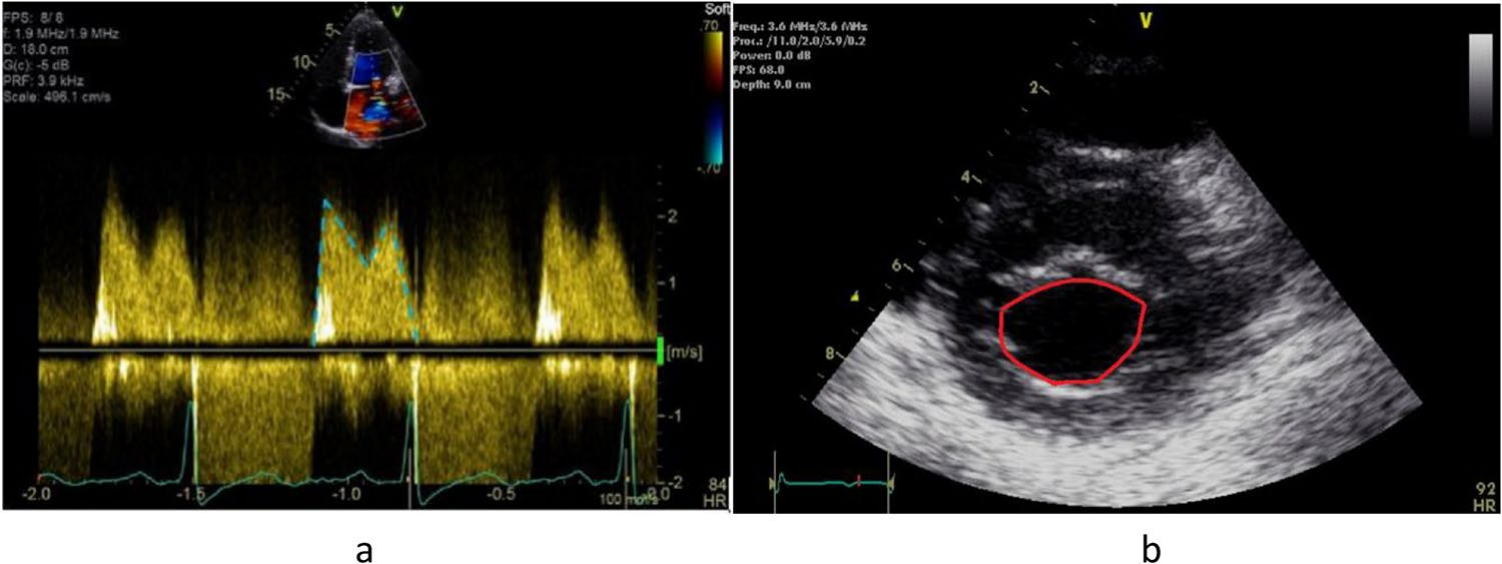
a Mitral inflow Doppler. Continuous wave Doppler measurement of TMPG (blue/green-dashed line) from the apical 4-chamber view. b Technique for estimating mitral valve area. Two-dimensional planimetry (red circular line) from a parasternal short-axis view was performed during diastole
